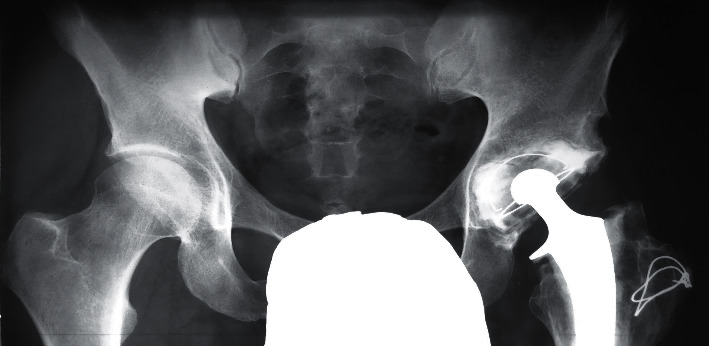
X-ray after primary total hip replacement (1975).
